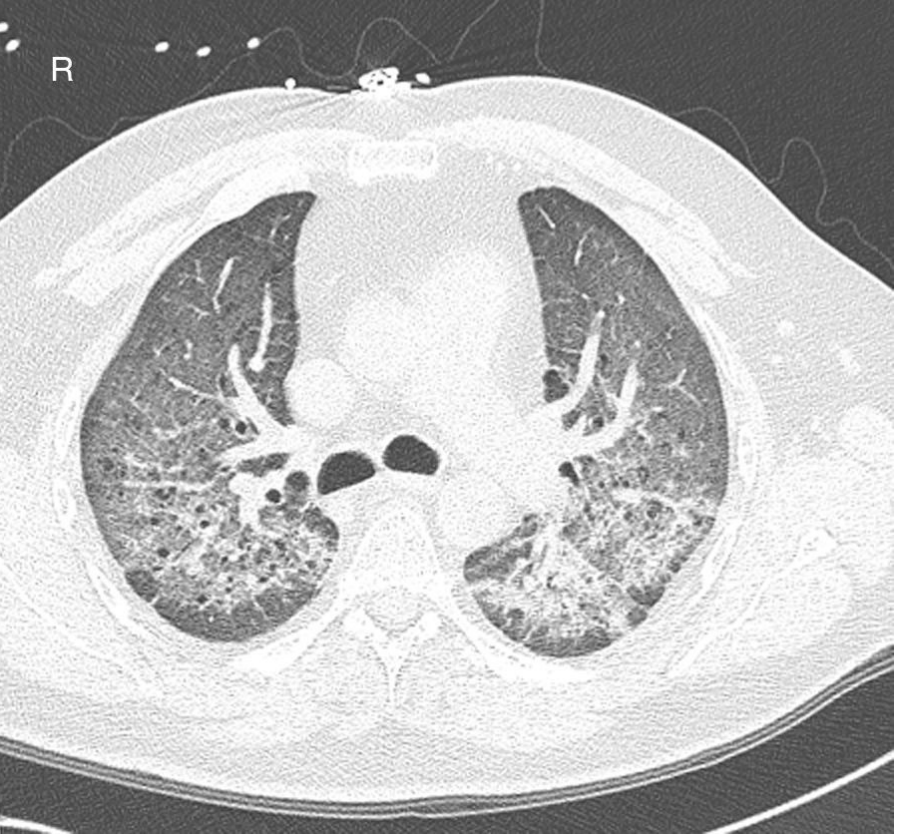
Computed tomographic (CT) scan. Non-contrast CT scan showing diffuse bilateral ground-glass opacities.
